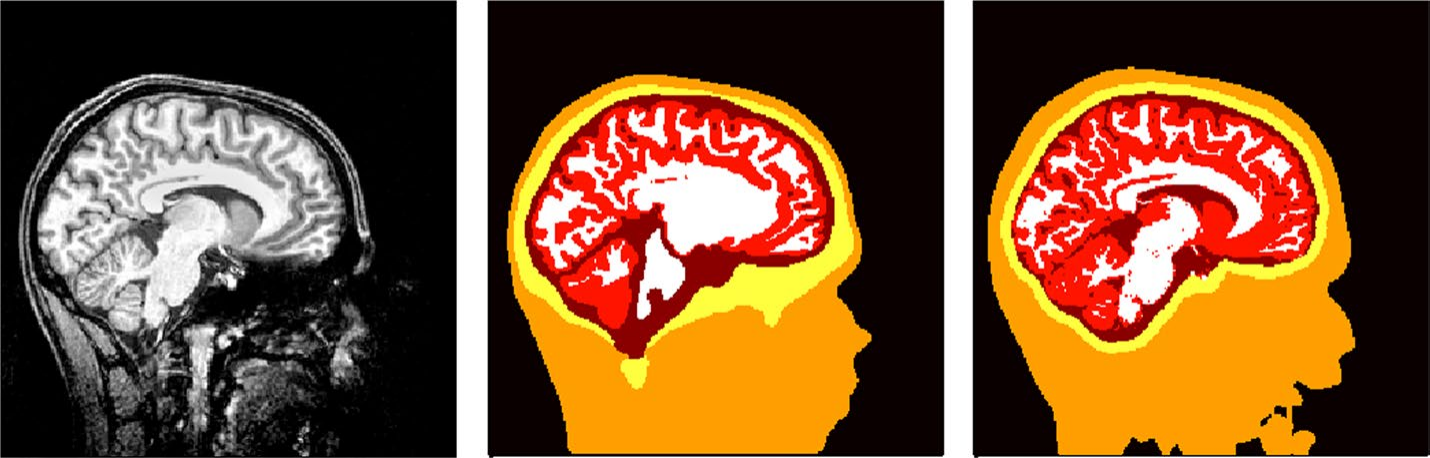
Original MRI (left), manually corrected segmentation (middle), and automatically generated segmentation using FieldTrip (right)
